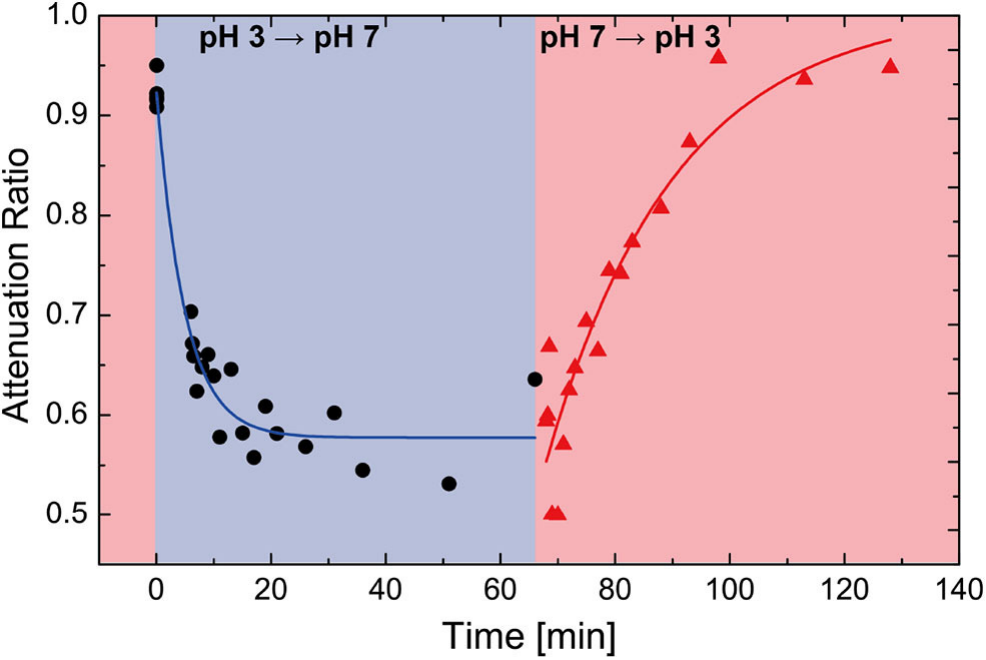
Time evolution data of the ultrasonic hydrogel pH sensor. The shaded background indicates the pH of the buffer solution that the sensor is embedded in red: pH 3, blue: pH 7.
